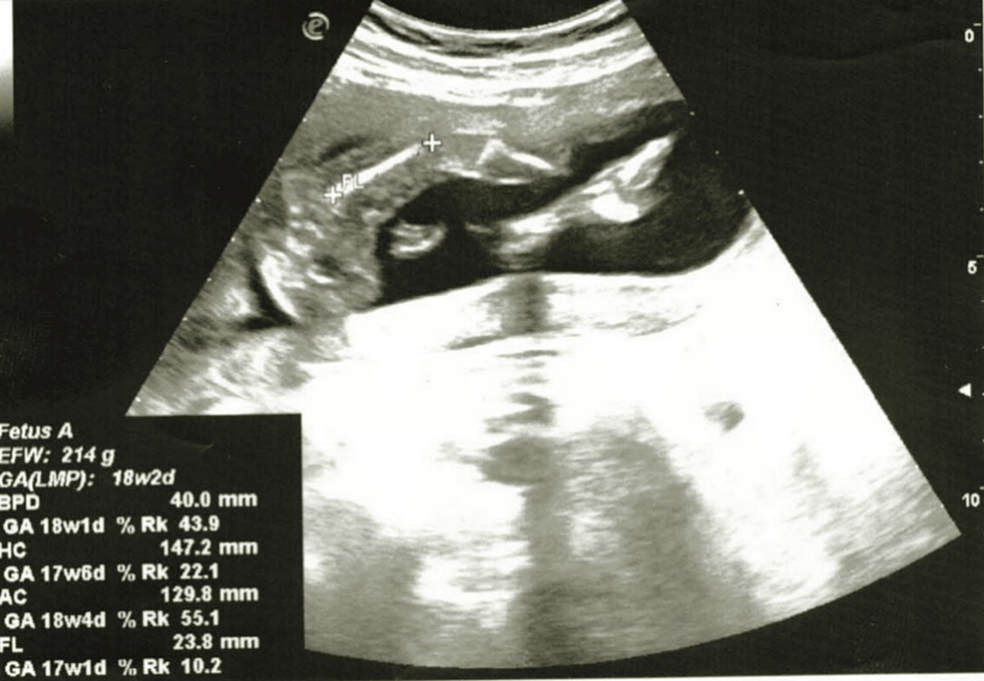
Ultrasound picture of the FL: 23.8 mm (reference limits: 18-32 mm) FL, femur length
